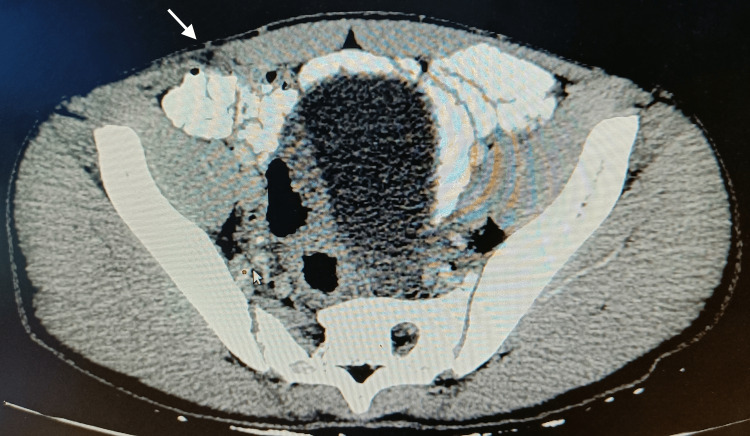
Contrast-enhanced CT abdomen cross-sectional view shows a defect in the Spigelian fascia lateral to the right rectus abdominis muscle indicated by a white arrow
